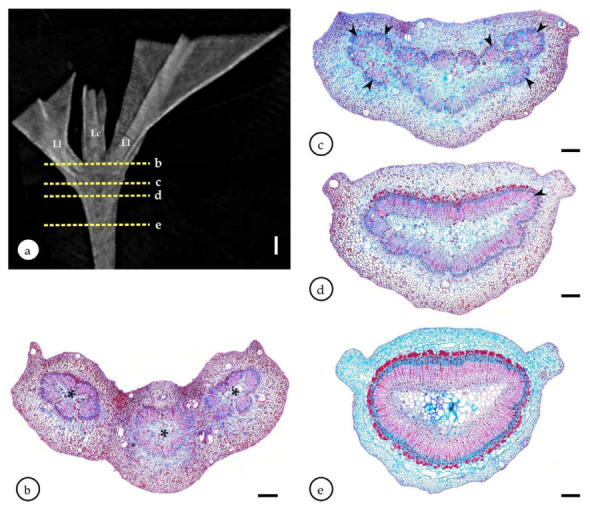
Section sequentially showing the vascularization at the insertion point of the lateral leaflets in the trifoliolate pattern of *Galipea jasminiflora*. (a) General view of the petiole of the trifoliolate compound leaf in micro–CT. (b–e) Transverse section from the distal to the proximal of petiole. (b) Insertion point of the three leaflets (*). (c,d) Traces of the lateral leaflets integrating into the axial system of the central leaflet (arrows). (e) Vascular system organized into a closed vascular cylinder. Lc = leaflet central; Ll = lateral leaflets. Scale bars: (a) = 100 μm; (b–e) = 200 μm.
